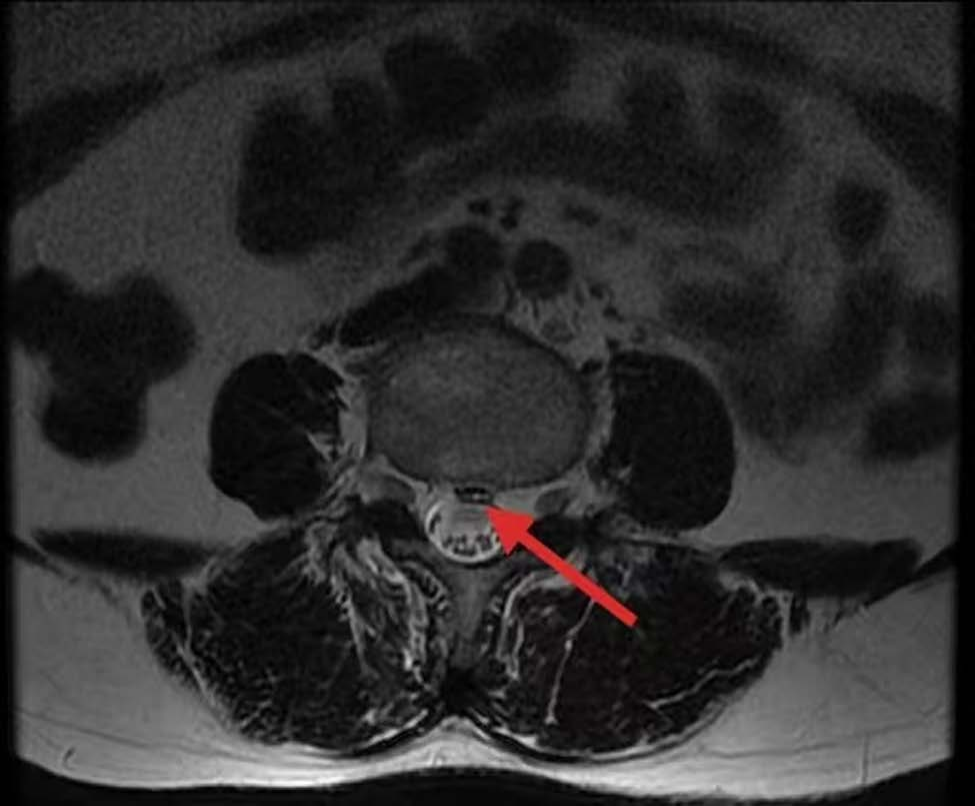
The red arrow in the MRI image indicates the compressed spinal cord. MRI = magnetic resonance imaging.
